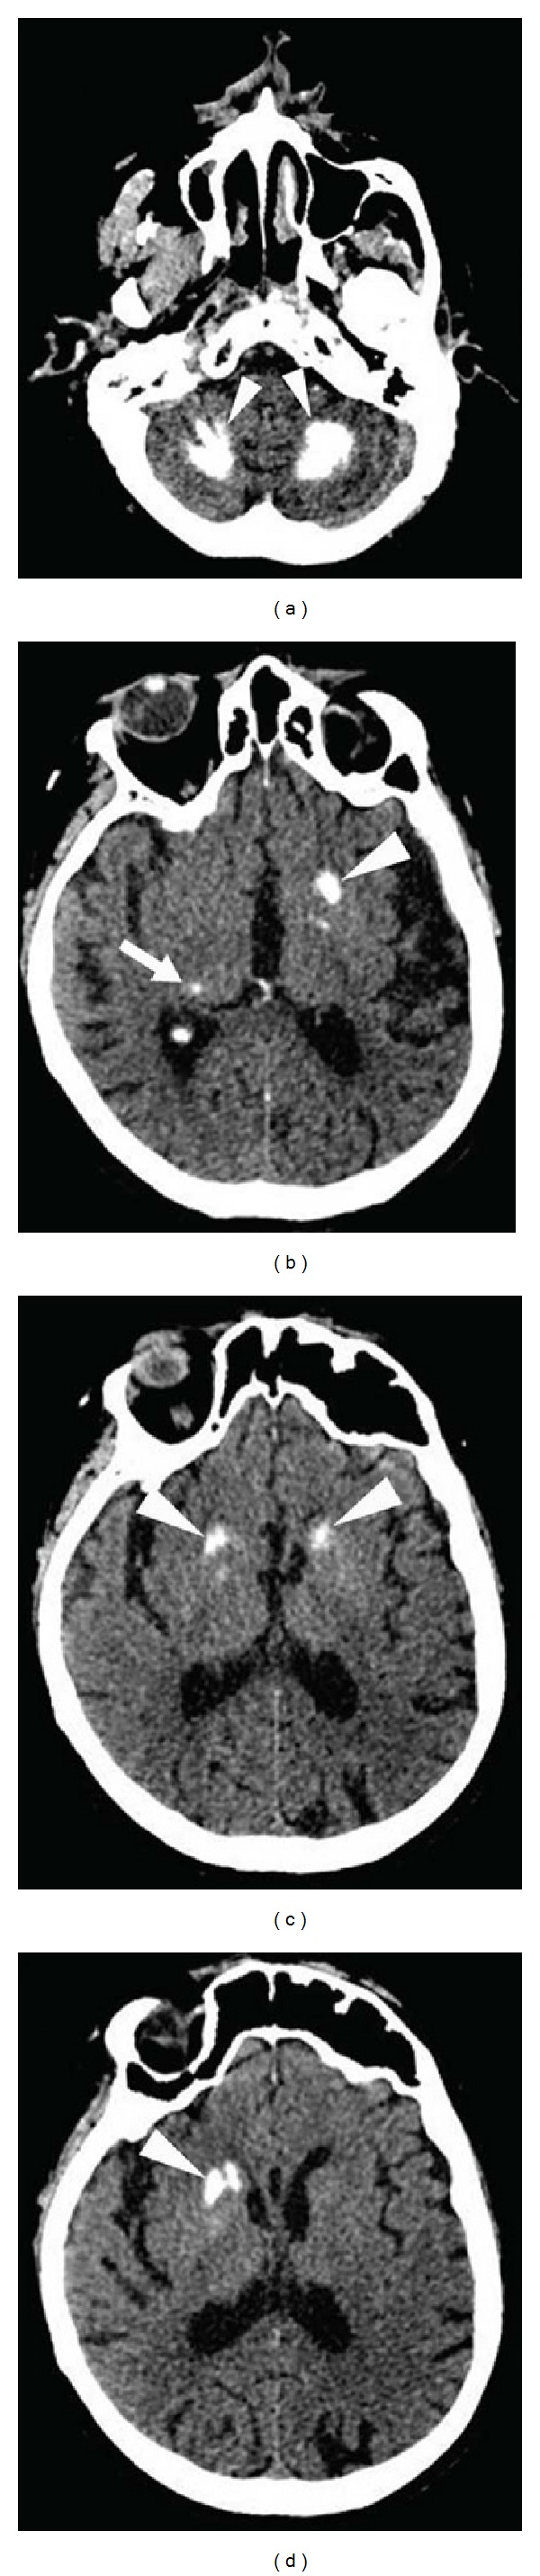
A patient with vitamin D deficiency with extensive cerebellar (a) and basal ganglia (arrowheads on b, c, and d) and thalamic (arrow on b) calcifications on CT.
